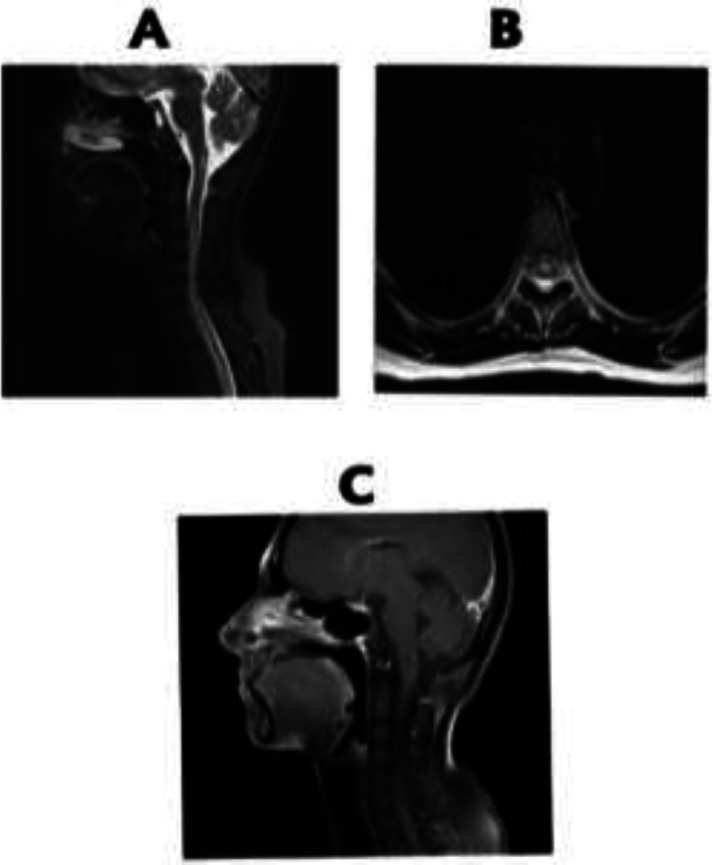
A. Cervical MRI revealed longitudinal extensive transverse myelitis (LETM) B. axial view showed central involvement of cervical cord. C. Cervical MRI with gadolinium revealed ring enhancement
